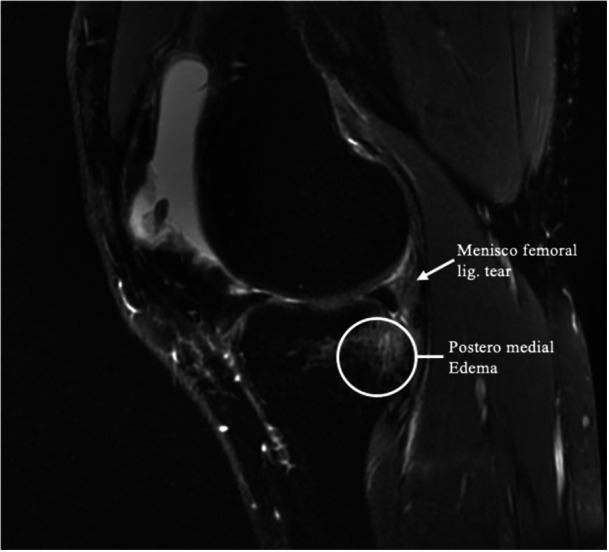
Magnetic resonance imaging (MRI) view: posteromedial oedema and meniscofemoral ligament tear.
